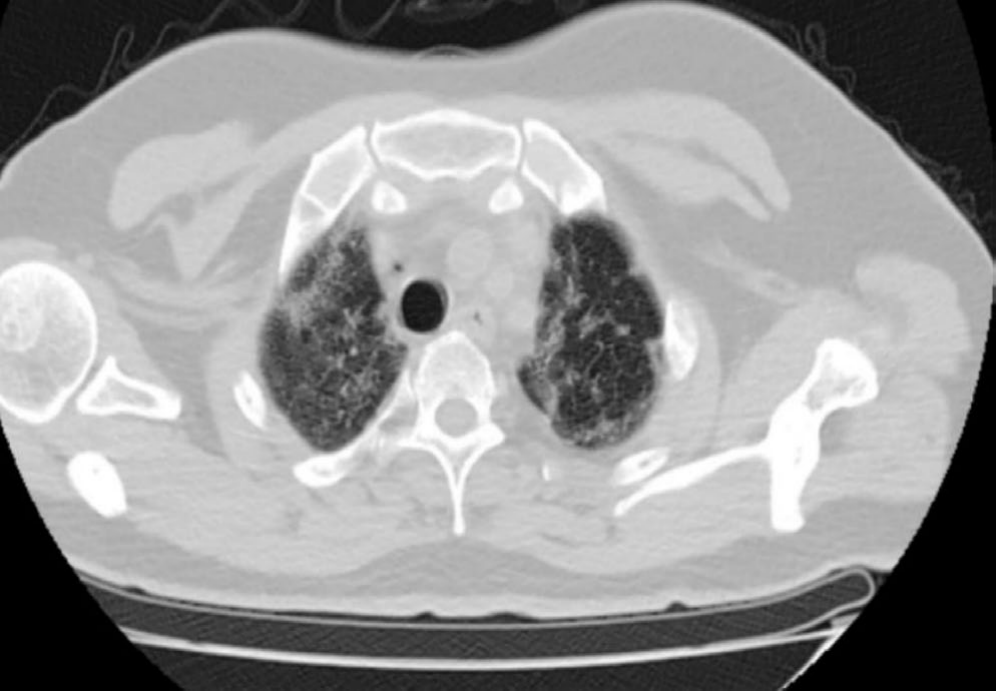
Follow‐up non‐contrast chest CT on lung window at the level just above the arch of the aorta shows bilateral patchy consolidation with interlobular septal thickening with reticulation and architectural distortion demonstrating early post‐COVID‐19 fibrotic changes. It also shows resolution of the pneumomediastinum and subcutaneous emphysema.
